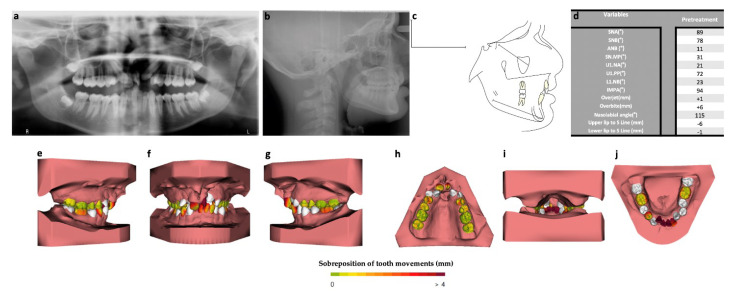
Pretreatment records: (a) orthopantomography; (b) lateral cephalogram; (c) cephalometric analysis; (d) dental casts; (e) right side; (f) frontal side; (g) left side; (h) superior arch; (i) back side; (j) inferior arch.
